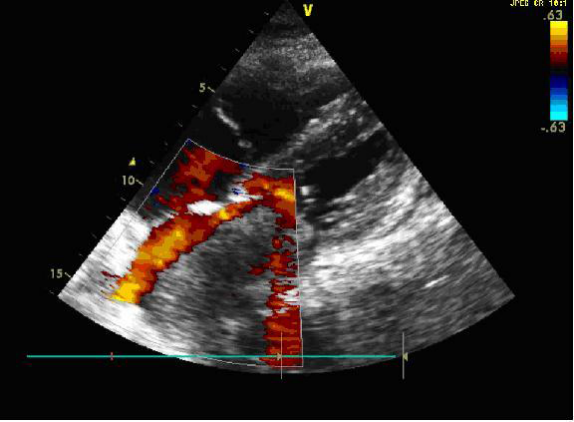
Echocardiogram with a left atrial mass protruding through the mitral valve.
